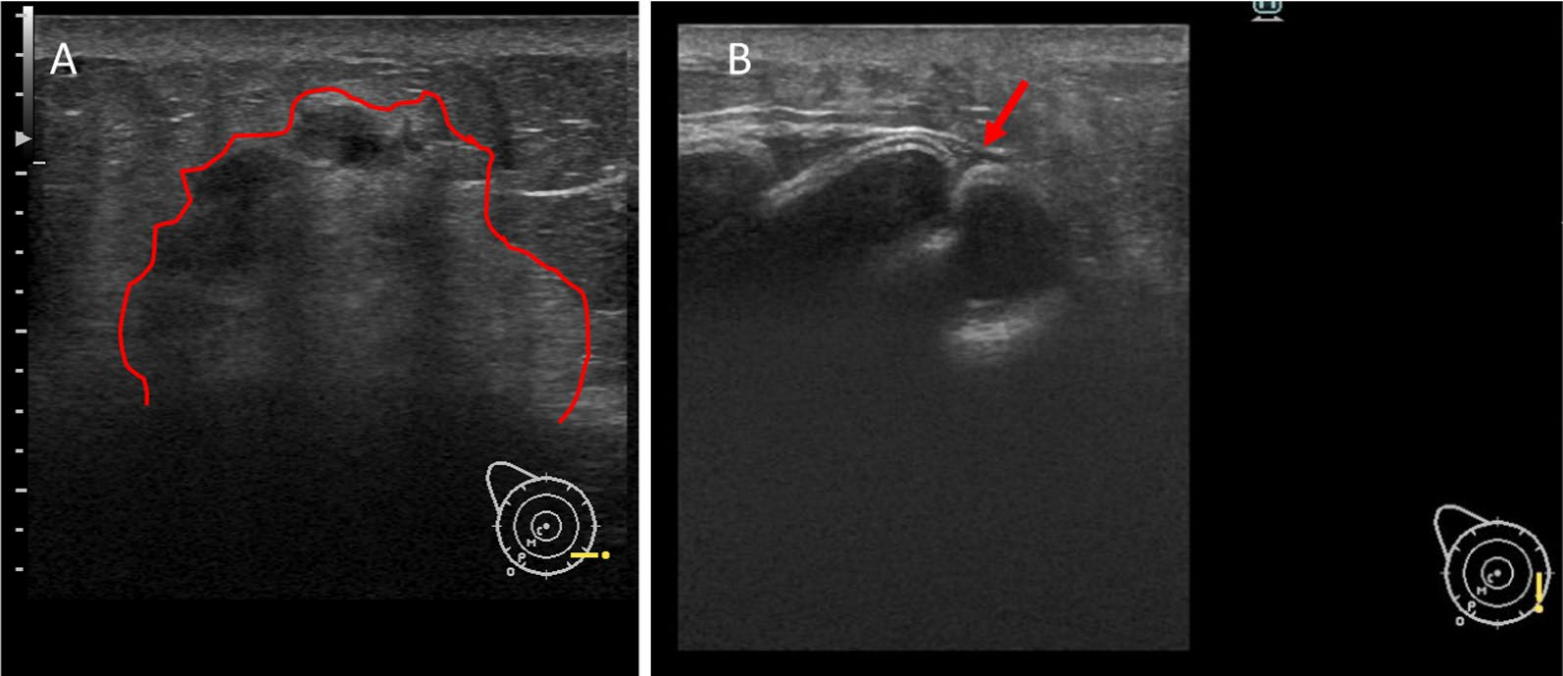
Ultrasound findings. A A hypoechoic irregular mass with a surrounding hyperechoic area was recognized in the inner inframammary area of the right breast. The outline is shown in red line B. The implant shell was deformed and a wavy pattern was recognized (red arrow)
